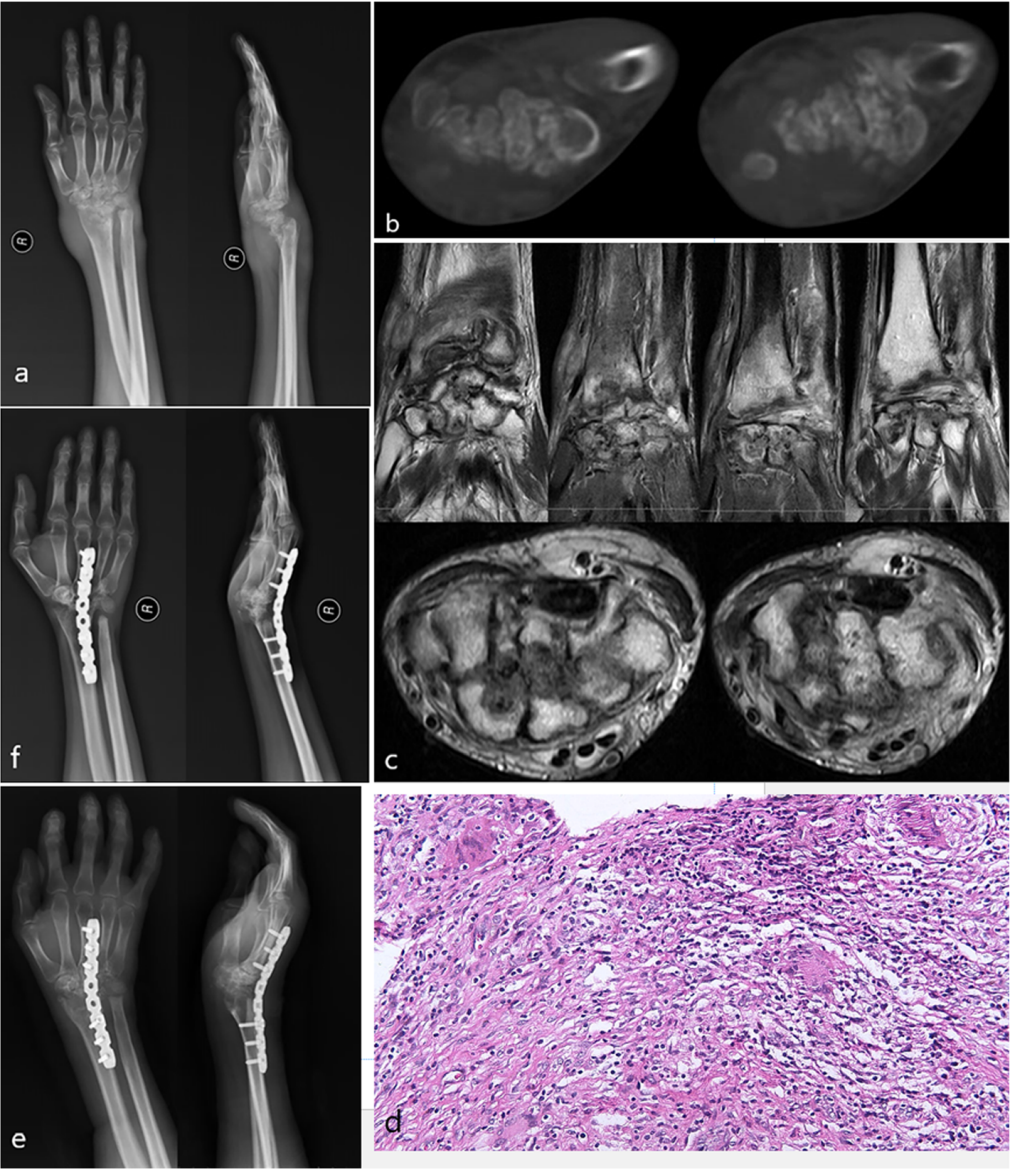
a Conventional radiography anterior posterior and lateral view pre-operation, b CT scan pre-operation, c pre-operative MR images, d the biopsy pre-operation, e X-ray scan 1 month post-operation, f X-ray scan 48 months post-operation
